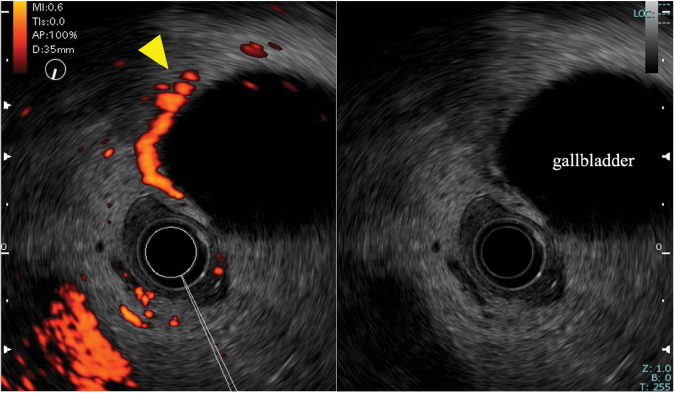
Endoscopic ultrasonography findings. Endoscopic ultrasonography revealed prominent blood flow around the gallbladder neck suggestive of varices (yellow arrow).
